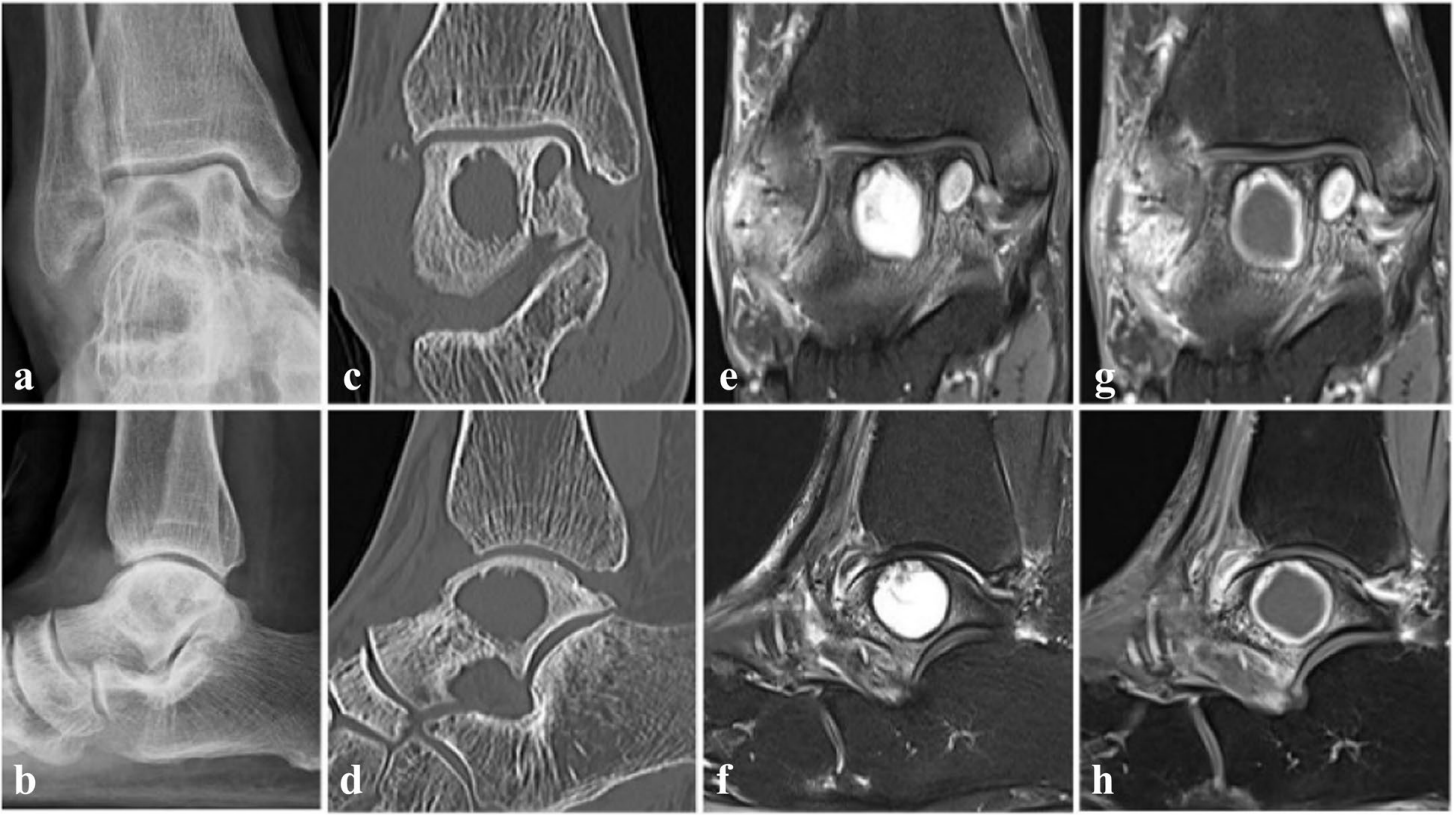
Plain radiograph, CT and MRI after recurrence. Plain radiographs in anteroposterior and lateral view (a, b) showing multiple huge osteolytic lesions of talus. CT scan in coronal and sagittal view (c, d) showing large osteolytic lesions with sclerotic rim yet without talar roof collapse. Proton density and T2-weighted images with fat suppression view (e, f) revealed cystic abscess of talus with sclerotic rim and surrounding bone marrow edema. Wall enhancement of the cystic lesions with Gadolinium contrast was observed (g, h)
